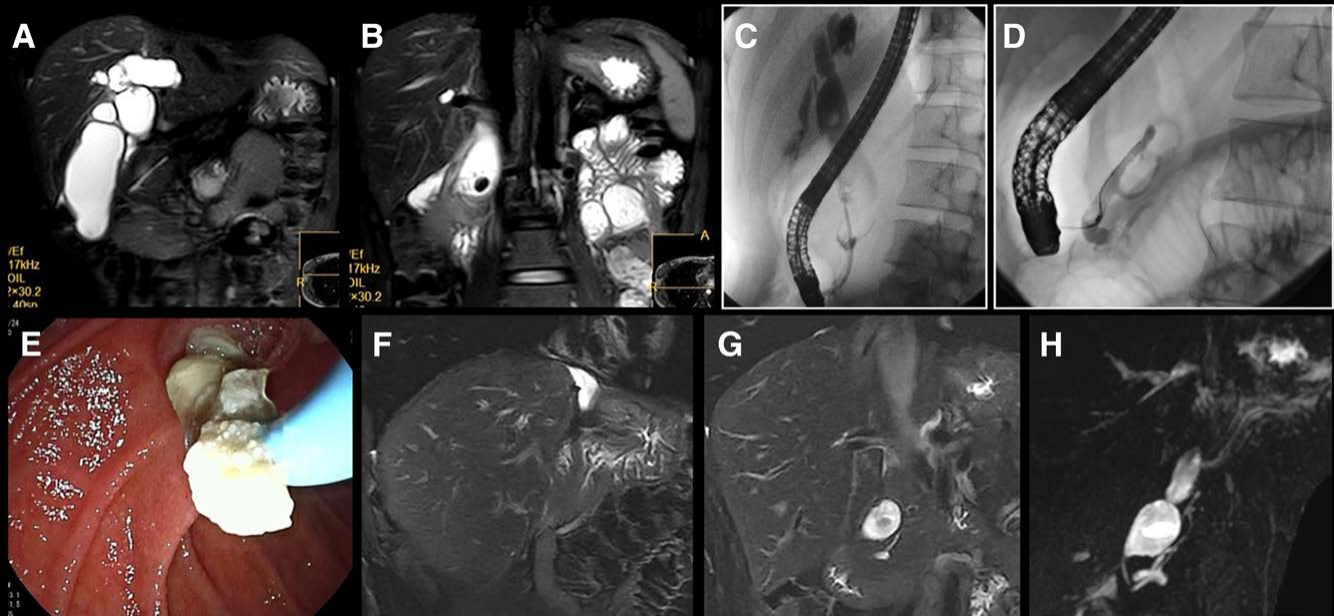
Treatment outcomes and follow-up process: (A) MRI in November 2020 showing dilation of the common bile duct and left hepatic duct. (B) MRI in November 2020 revealing the original common bile duct stone. (C) ERCP cholangiogram in November 2020. (D) ERCP accessory pancreatic ductogram in November 2020. (E) Partial removal of pancreatic duct stones during ERCP in November 2020. (F) MRI in April 2024 showing encapsulated effusion in the left lobe of the liver. (G) MRI in April 2024 revealing residual dilation and stones in the lower segment of the common bile duct. (H) MRCP in April 2024 showing no significant dilation or stones in the pancreatic duct. ERCP = endoscopic retrograde cholangiopancreatography, MRCP = magnetic resonance cholangiopancreatography.
